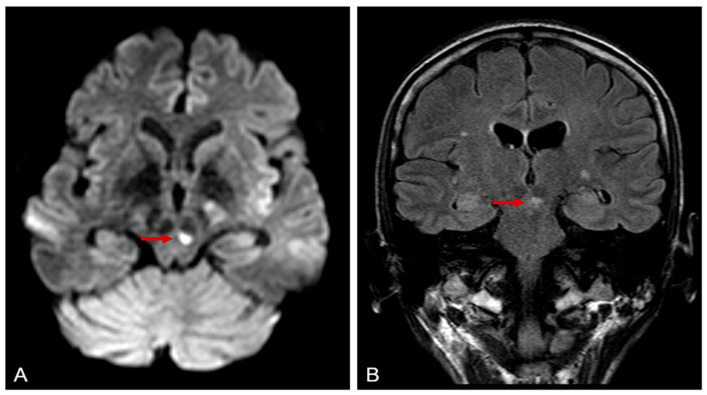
Brain magnetic resonance imaging (MRI). (A) Diffusion-weighted axial image (DWI) and Fluid Attenuated Inversion Recovery (FLAIR) (B) coronal image demonstrate hyperintense lesion in the mesencephalon, consistent with an acute ischemic lesion.
